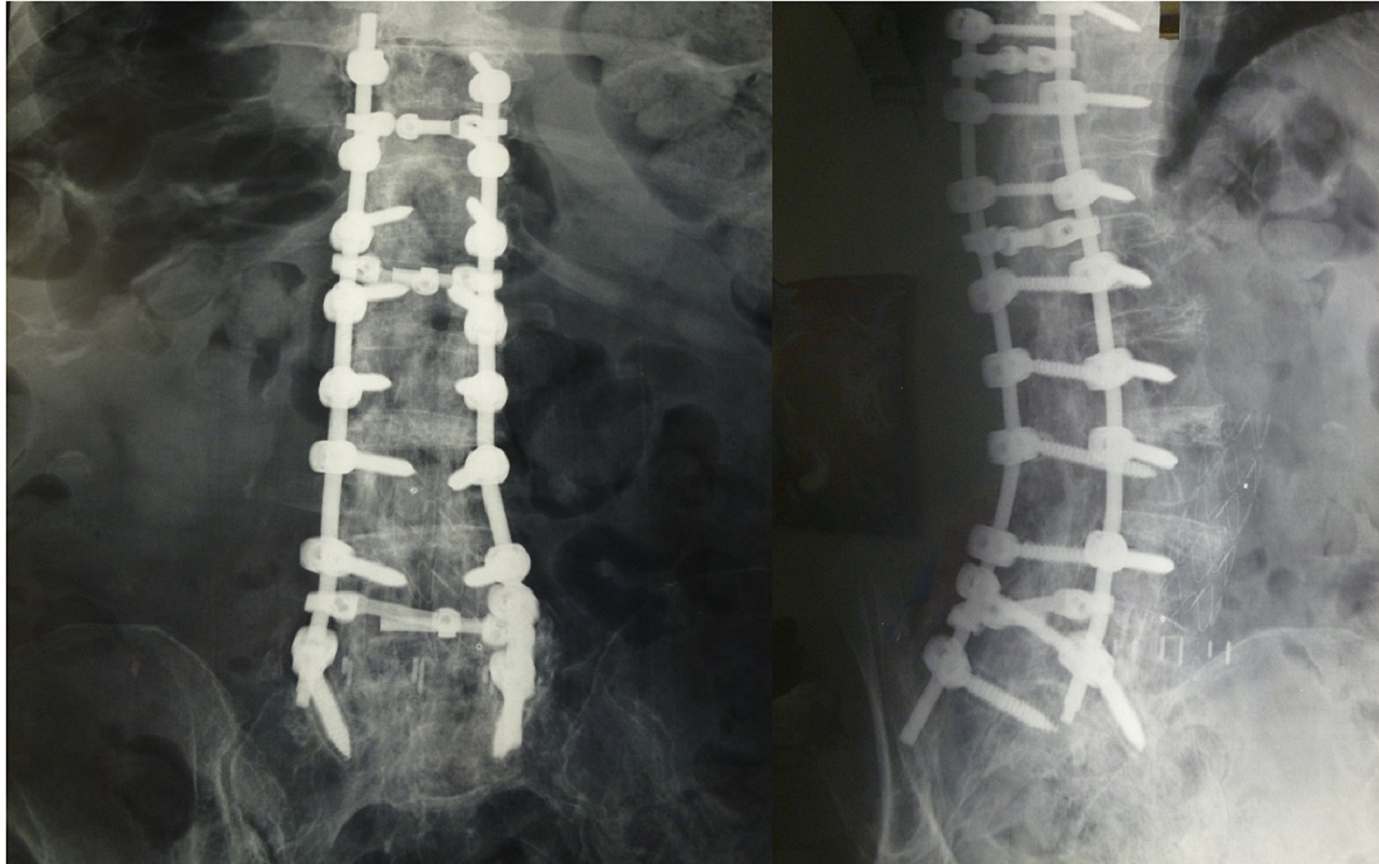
X-ray imaging (AP and Oblique views) of the lower thoracic and entire lumbar spine shows posterior spinal fusion at the level of T10-S1
